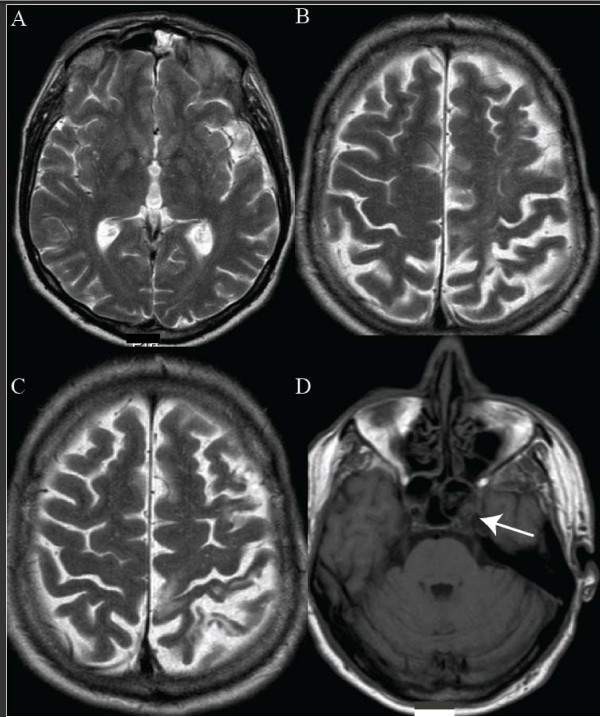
Atrophy of the left frontoparietal lobe, with extensive gliosis (A, B, C; T2-weighted MRI). The left internal carotid artery is occluded, since there is no flow void (D; T1-weighted MRI).
